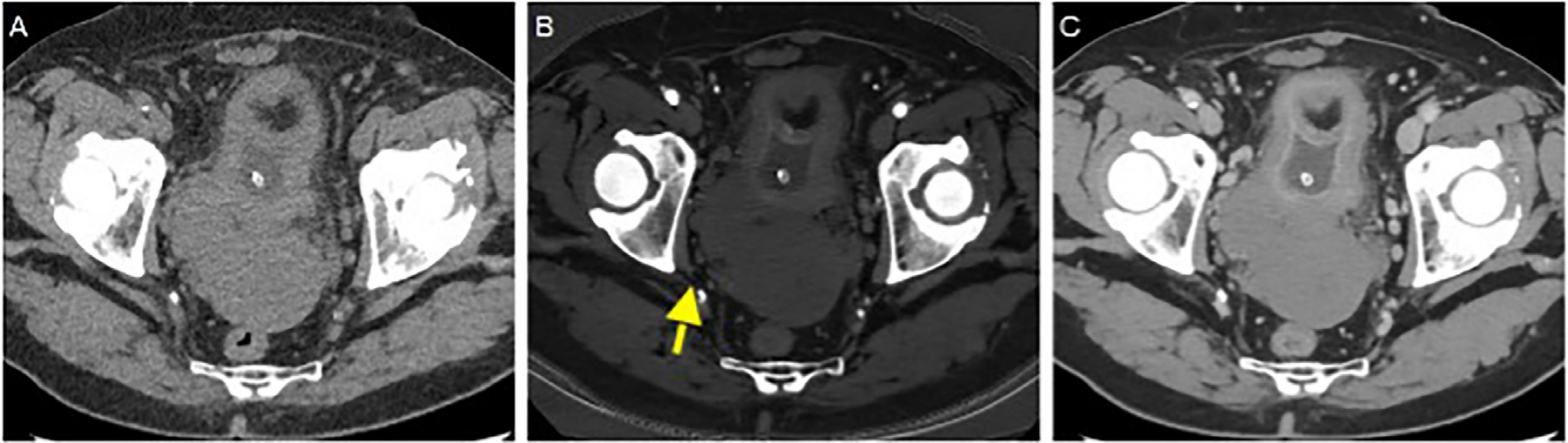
Axial non contrast (A), arterial Dual Energy Iodine Water (B, arrow) and portal phase contrast (C) pelvic computed tomography, show a voluminous, polylobulated, solid mass with smooth margin (yellow arrow), between bladder (with a catheter) and rectum in the median-paramedian bilateral area but prevalent on the right, well-limited, with regular margins, homogeneously hypodense and with mild contrast enhancement prevalent in portal phase.
